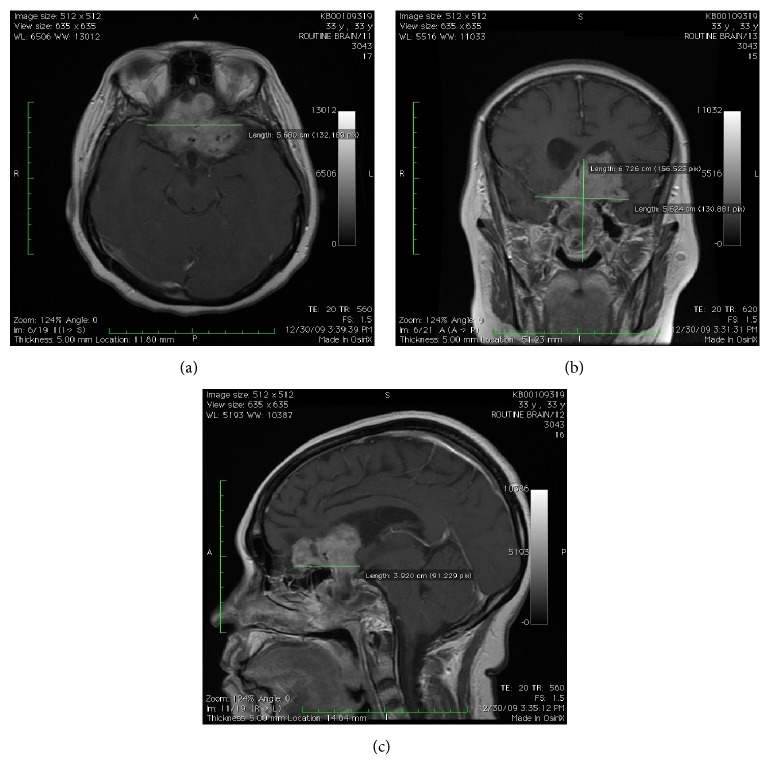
Contrast enhanced axial (a), coronal (b), and sagittal (c) T1-weighted sequence on MRI at 12-month follow-up showing reduction in size of the pituitary macroadenoma following treatment with cabergoline.
